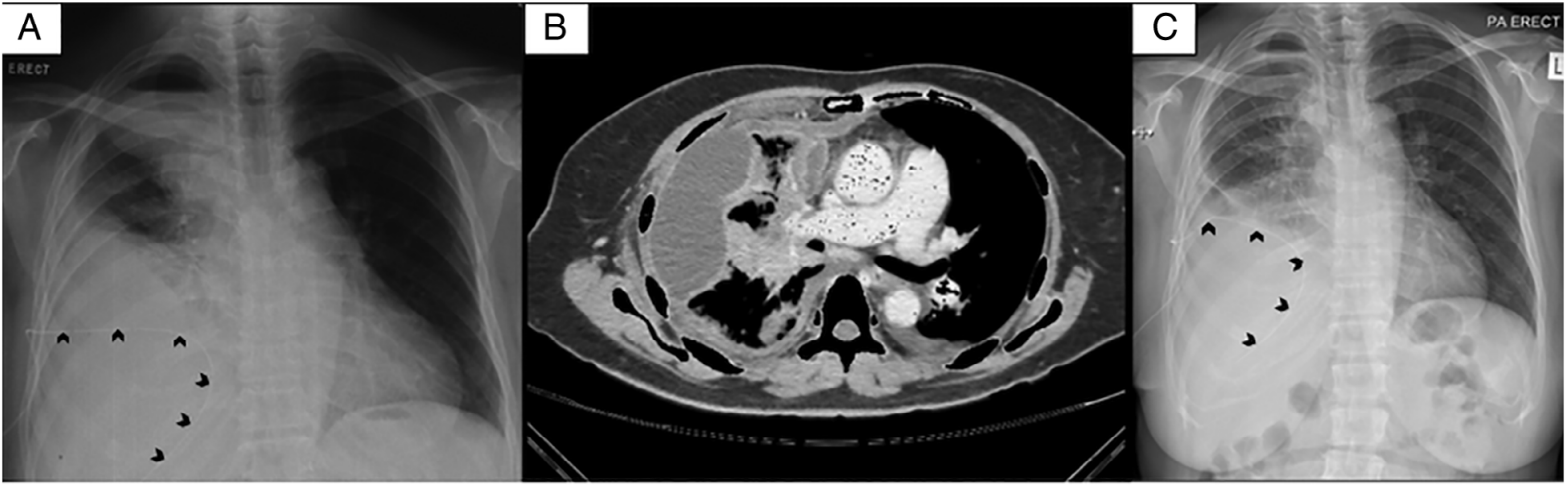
Chest radiograph (A) showed a loculated right pleural effusion with indwelling pleural catheter (IPC) in situ (black arrows). Computed tomography (CT) of the thorax (B) showed a loculated right pleural effusion. Chest radiograph (C) post IP alteplase showed improvement with minimal residual pleural effusion and IPC in situ (black arrows) with elevated right hemidiaphragm.
